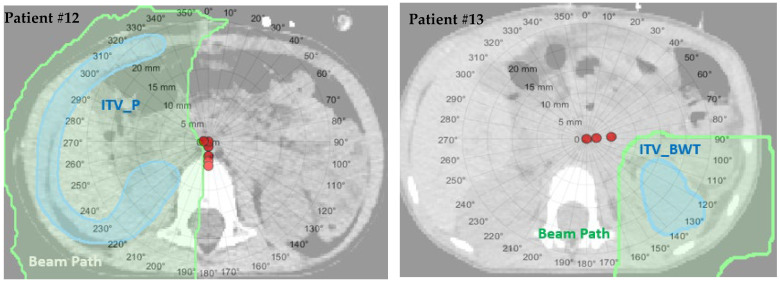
Polar plots, mapped in function of gantry angle and ΔWEPL (red data points), overlaid on axial CT images for thirteen Wilms’ tumor patients, highlighting the beam paths (20% isodose lines) in green shaded region and target volumes in blue and yellow.
